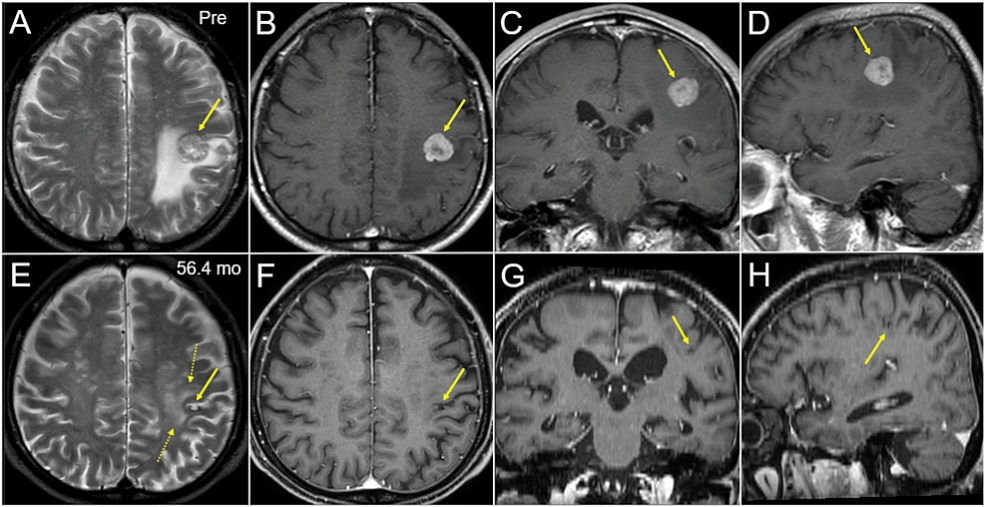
Magnetic resonance images before and 4.7 years after stereotactic radiosurgery. The images show T2-weighted images (WI) (A, E); contrast-enhanced (CE) T1-WI (B-D, F-H); axial images (A, B, E, F); coronal images (C, G); sagittal images (D, H); before stereotactic radiosurgery (SRS) (Pre) (A-D); and at 56.4 months (mo) after SRS (E-H). (A-H) All images were co-registered on MIM Maestro^TM^ software (MIM Software Inc., Beachwood, Ohio, United States) and are shown in the same magnification and coordinates; (A-D) A solid mass lesion (arrows in A-D) in the left frontoparietal lobes is concomitant with surrounding edema almost not extending into the ventral lobe; (E-H) The tumor remnant is observed as a cavitary lesion (arrows in E-H) in the left postcentral gyrus; (E) A slight high-intensity change is seen in the surrounding white matter (dashed arrows in E); (E-H) Progression of brain parenchyma atrophy and relevant ventricular dilatation are seen.
